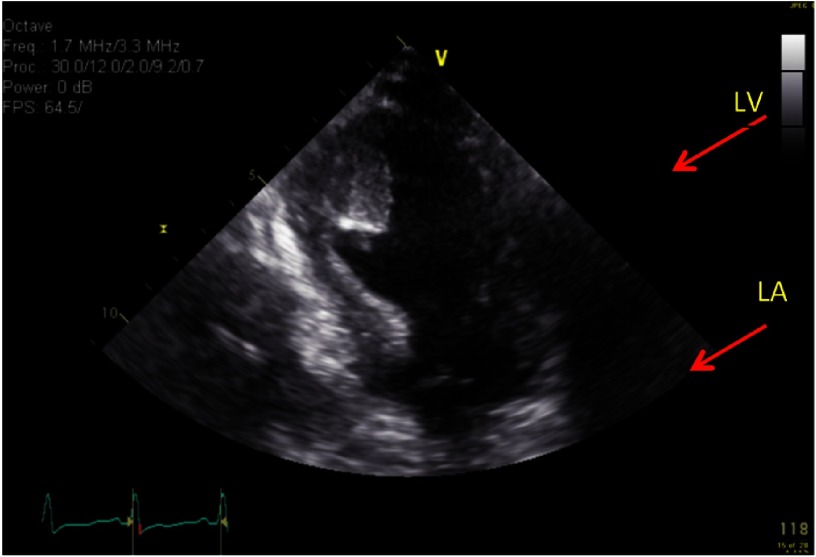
Apical two-chamber view showing thickened ventricular septum and apical clot.
